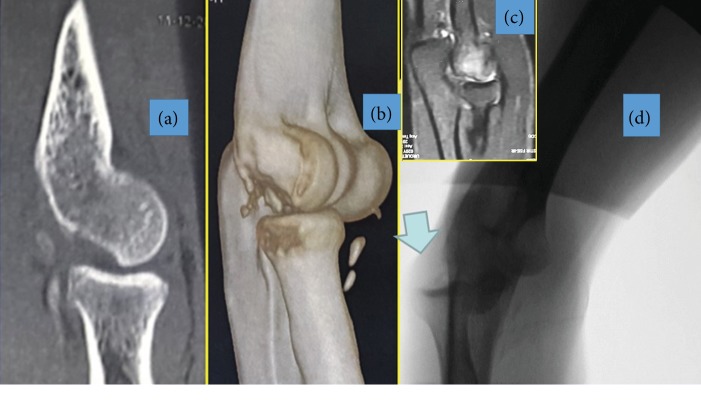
(a, b, and c) TC scan and MRI showing capitellum fracture. (d) Engaging pivot shift.
